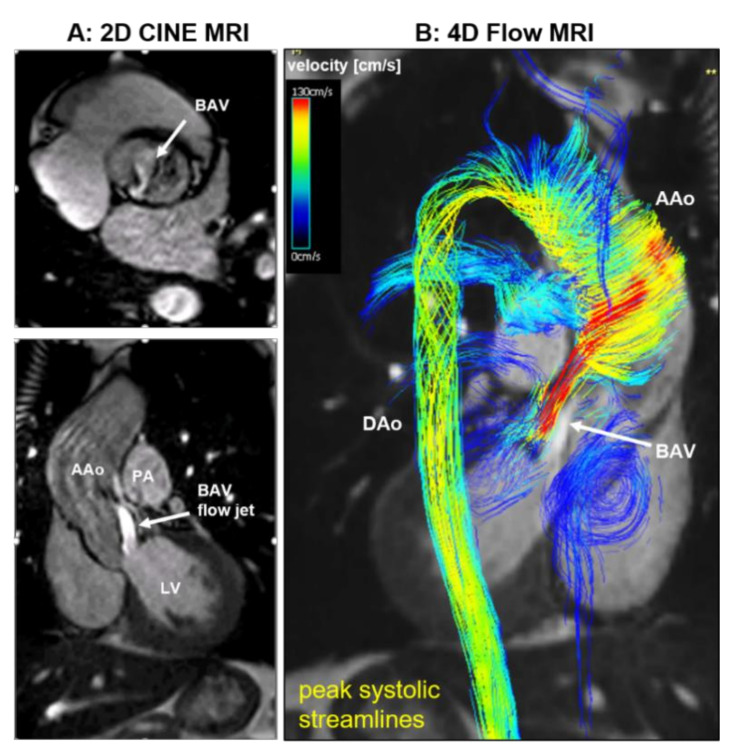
The 2D CINE (A) and 4D flow cardiovascular magnetic resonance (CMR) (B) in a 32-year old patient with bicuspid aortic valve (BAV, right-noncoronary (R-N) valve fusion pattern), aortic dilatation (Sinus of Valsalva/Mid Ascending Aorta diameter = 42/45 mm), moderate aortic valve stenosis (aortic valve area = 1.5 cm^2^). A: 2D CINE MRI during peak systole shows limited BAV opening (top) and the associated outflow jet (bottom) B: 3D streamline visualization of systolic blood flow in the thoracic aortic as assessed by 4D flow CMR and shows a marked high-velocity (red color) valve outflow jet directed toward the anterior wall of the ascending aorta (AAo). Note the formation of a complex helix flow pattern in the entire AAo. In addition, 4D flow CMR provides full volumetric coverage of the thoracic aorta and flexible retrospective quantification of peak systolic velocities at multiple locations in the thoracic aorta. LV = left ventricle, PA = pulmonary artery, DAo = descending aorta.
